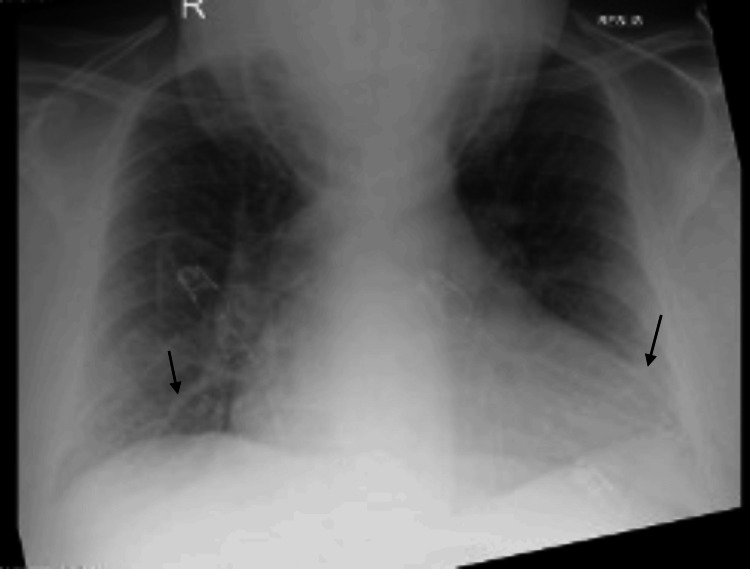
Initial chest X-ray demonstrating bibasal atelectasis (indicated by arrows)
